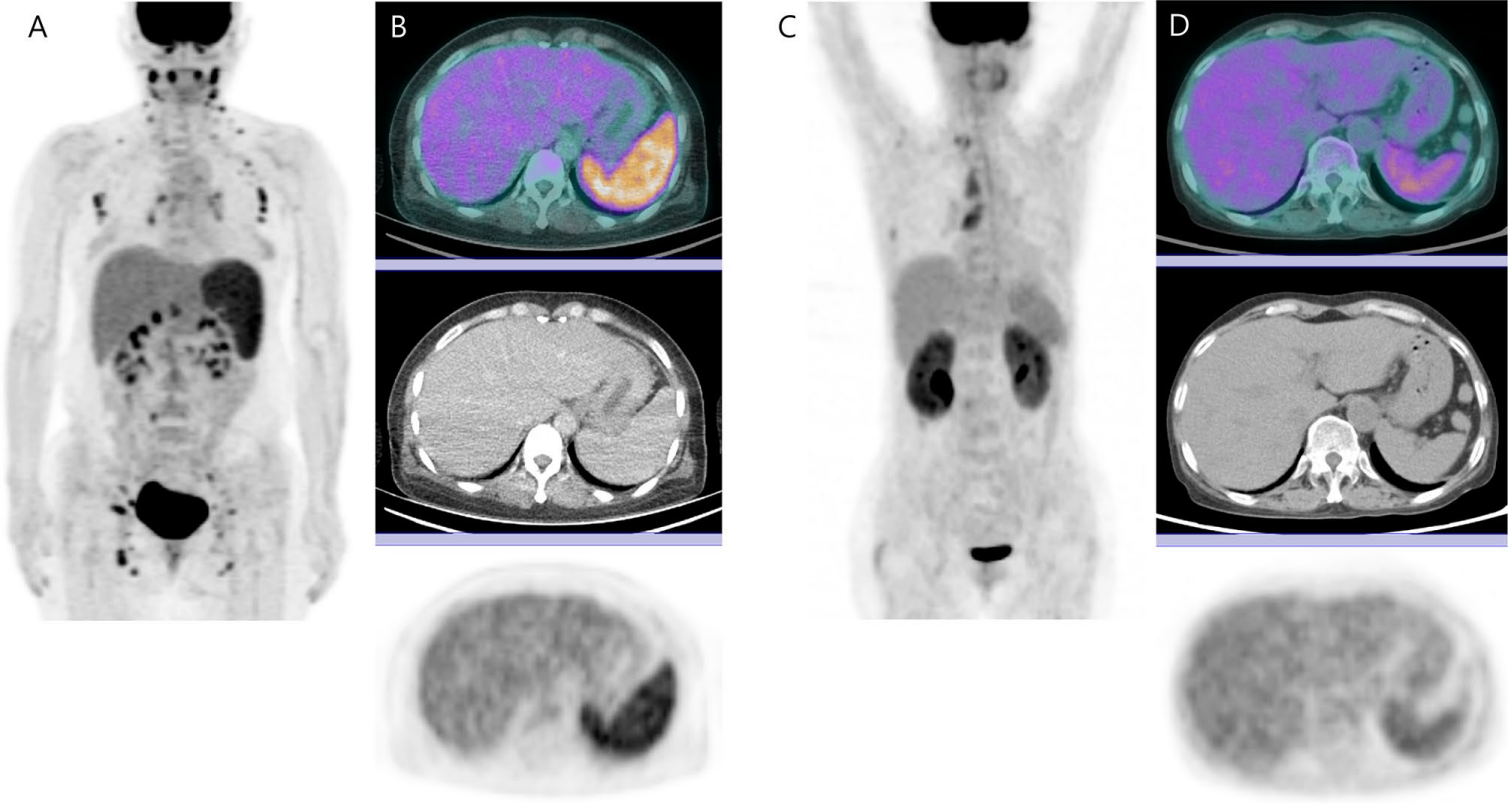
FDG PET/CT images of a 49 year-old female patient with scrub typhus and a 63 year-old female patient with SLE. The patient with scrub typhus demonstrates extensive involvement of lymph nodes from both cervical to inguinal areas (A), whereas the patient with SLE shows lymph nodes in the right supraclavicular and mediastinal areas (C). FDG uptake levels in the lymph nodes, spleen, and liver are higher in the patient with scrub typhus (B) compared to those in the patient with SLE (D).
